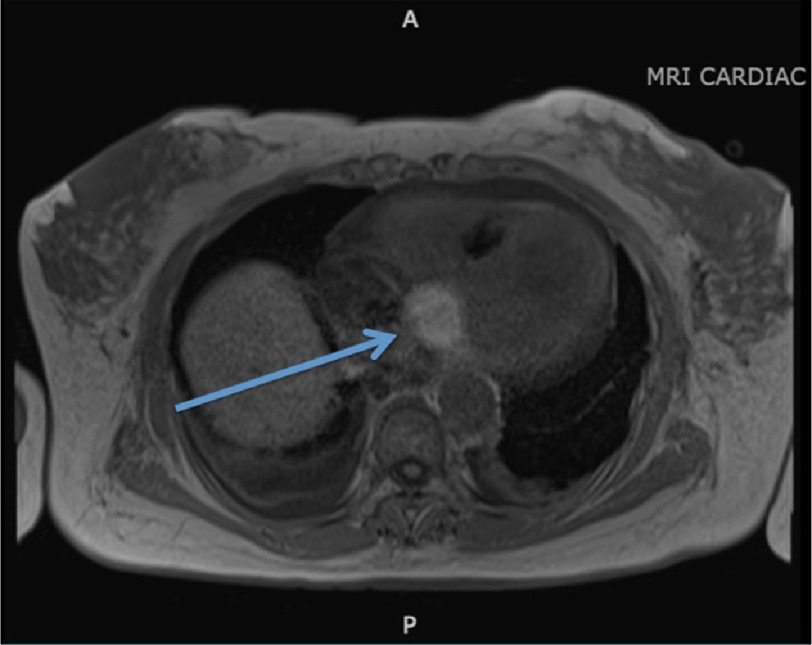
Axial slice of the patient’s cardiac magnetic resonance imaging (MRI). The arrow indicates the interatrial lesion centered within the region of the atrioventricular node.
